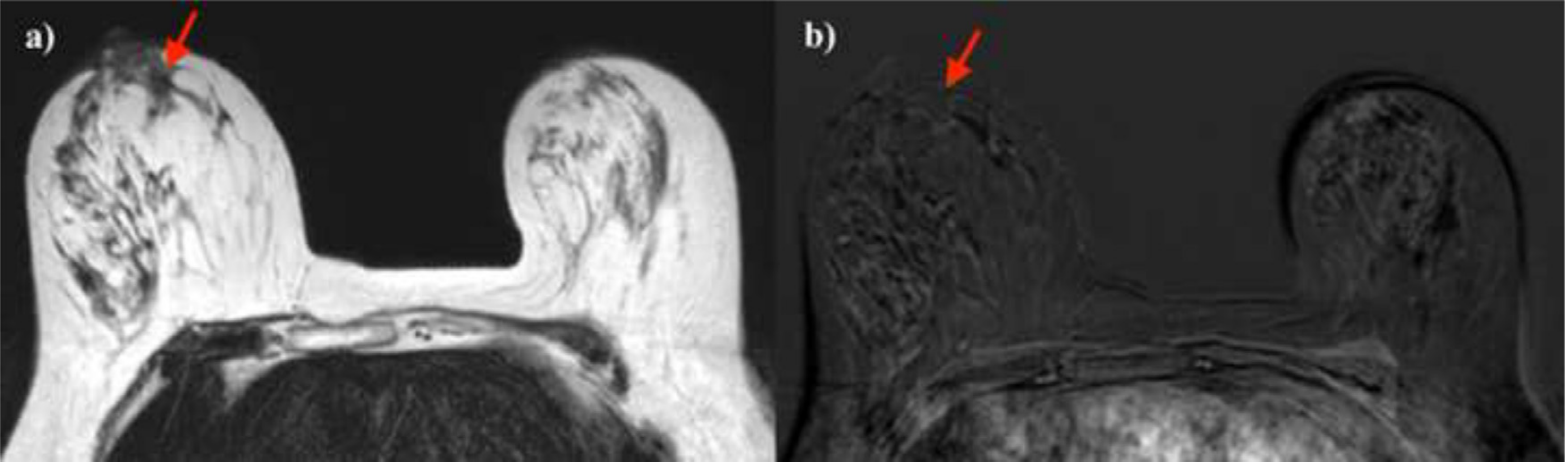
MR imaging after 21 days of treatment. T2-hypointense retroareolar area (A) with no significant contrast enhancement in fat-suppressed dynamic contrast-enhanced (DCE) images (B).
